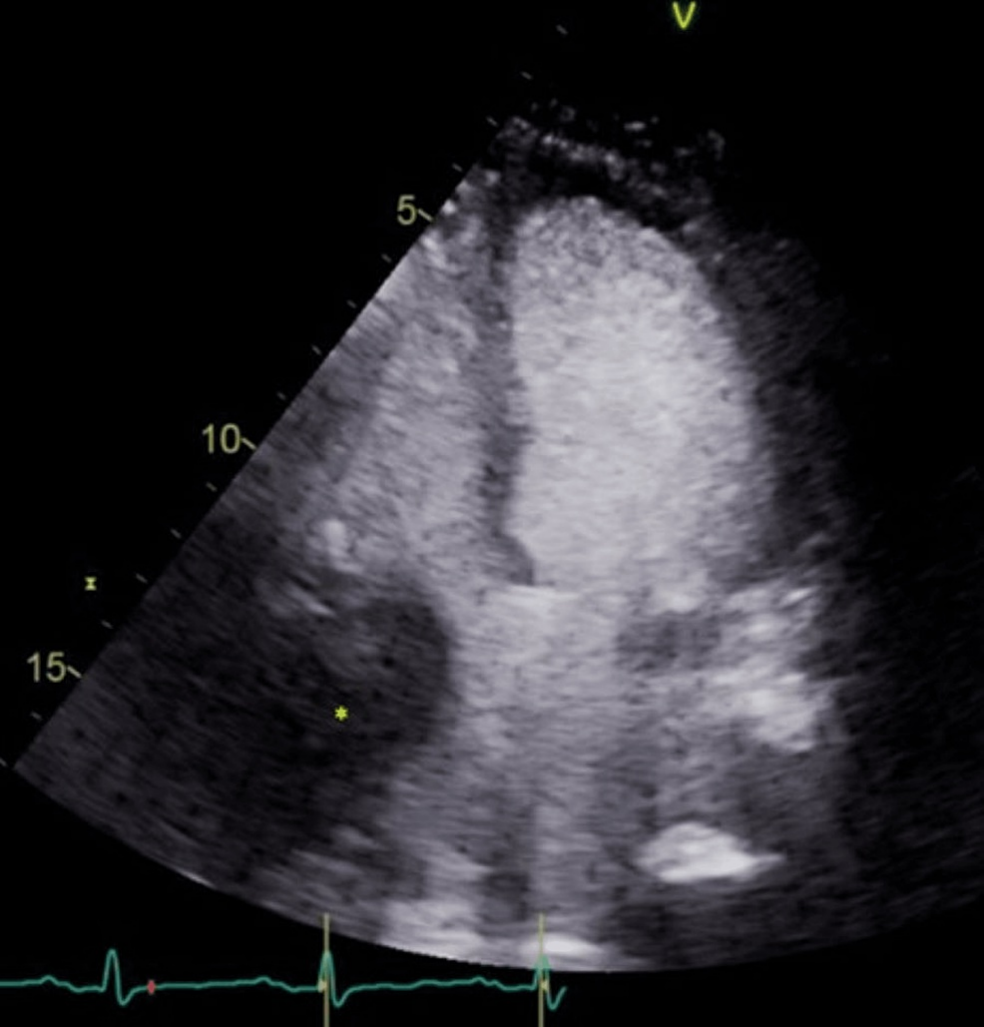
Transthoracic echocardiogram with the ultrasound-enhancing agent showing the right atrial mass (*).
